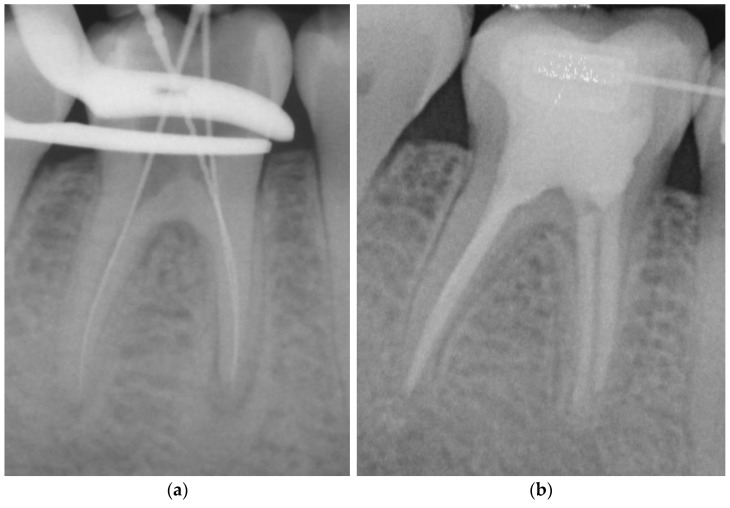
Representative root canal filling using the single-cone obturation technique with gutta-percha and GuttaFlow^®^ (SCGF); working length radiograph of tooth 46 (a); 6-year follow-up radiograph showing complete apical healing (b).
